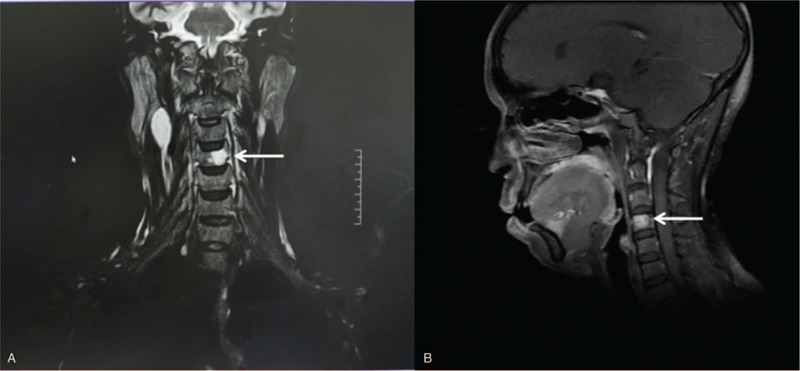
Neck magnetic resonance imaging (MRI). A, Neck MRI showing multiple and variously sized nodules in the right submandibular area with a slightly long signal on T1 or T2 and a hyperintense signal on DWI. B, Neck MRI showing the left margin of the C4 vertebral bone with a slightly long signal on T1 or T2 with bone destruction.
